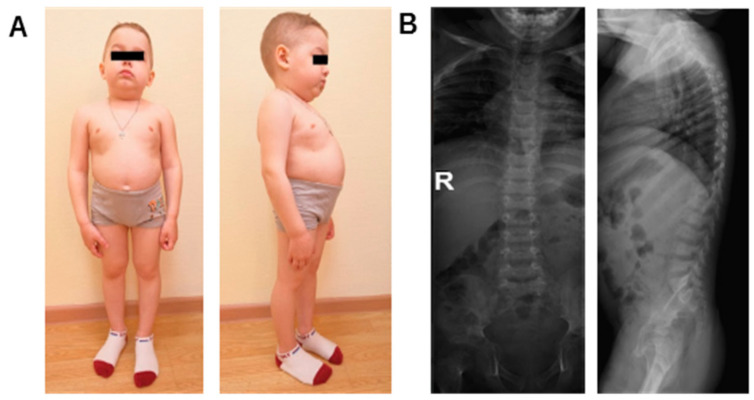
Common clinical features of patients with SIOD in our cohort. (A) Six-year-old boy with SIOD: short stature, disproportional short trunk, short neck, low auricles; (B) The spine X-ray of a child with SIOD. R—right side. In the lateral projection, the thoracic vertebrae are rounded, the anterior contours are smoothed, and the lumbar vertebrae are flattered. The height of the intervertebral spaces is significantly reduced in the thoracic region throughout and expanded in the lumbar region (due to deformation of the vertebral bodies).
